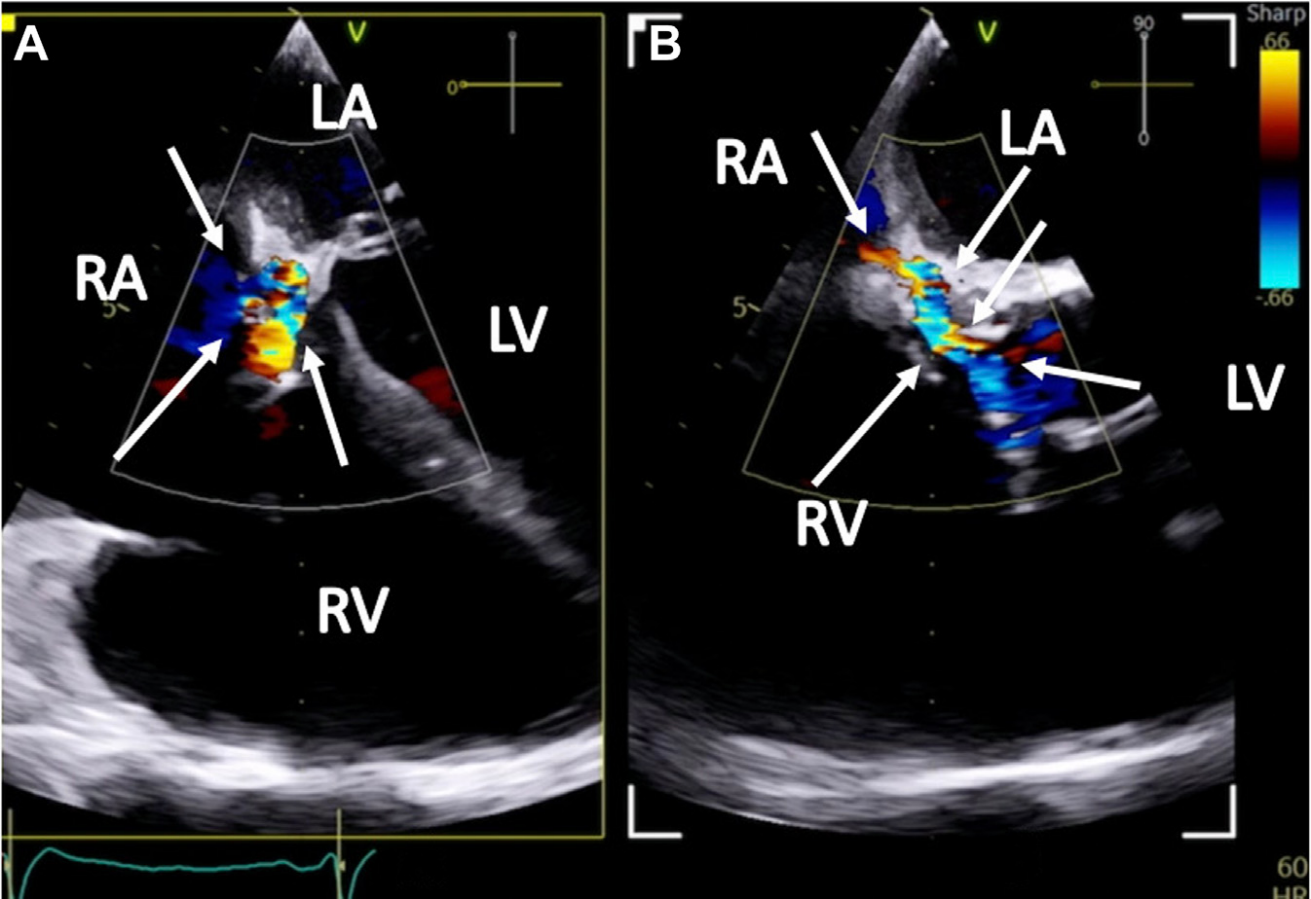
Transesophageal Echocardiographic Biplane Images at the Midesophageal Level With Color Flow Doppler (A and B) The arrows demonstrate abnormal flow between the aorta and the right atrium (RV; fistula between the aorta and the right atrium). Abbreviations as in Figure 2.
